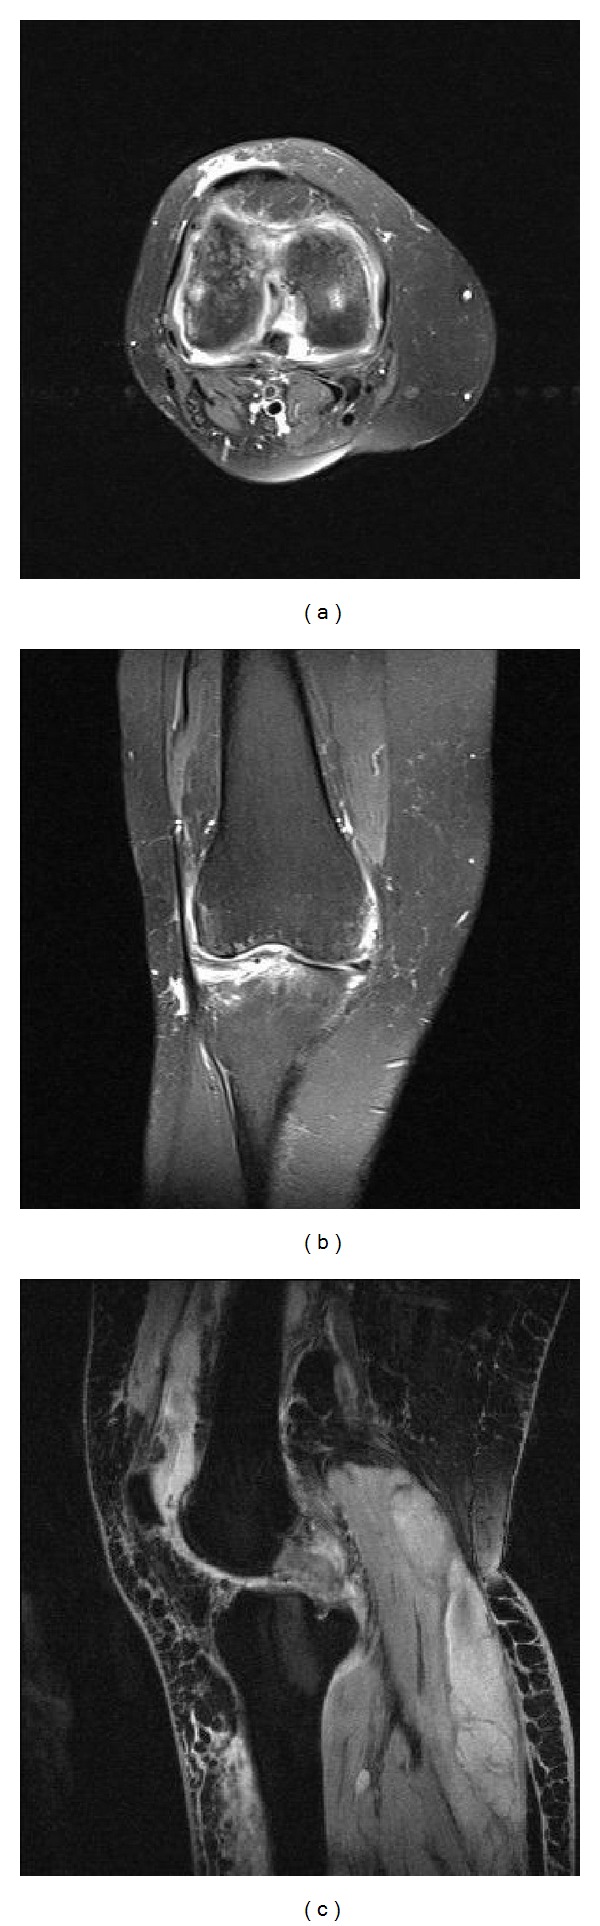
Postoperative T2-weighted MR images of the right knee, revealing effusion in the knee joint and popliteal fluid collection.
